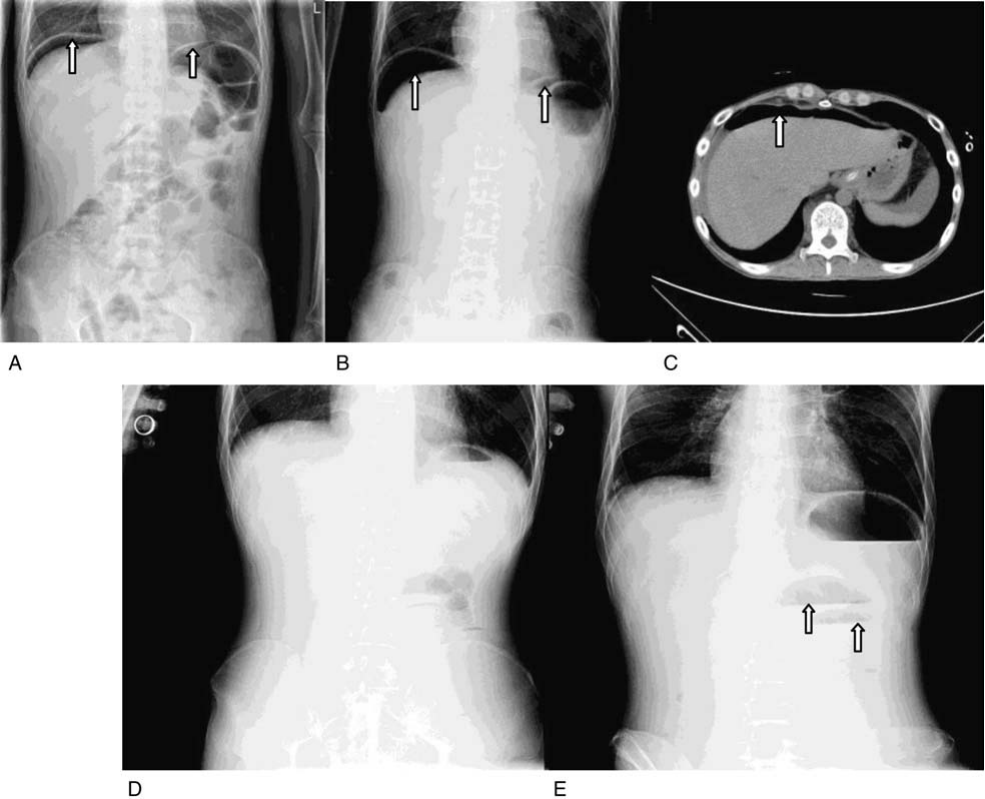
(A) An upright abdominal x-ray in a tertiary level hospital revealed bilateral moderate intraperitoneal free air. (B) An upright abdominal x-ray revealed bilateral massive intraperitoneal free air. (C) The image of abdominal CT showed free gas with a low diaphragm. (D) An upright abdominal x-ray did not show any free gas or liquid gas levels. (E) An upright abdominal x-ray showed visibly dilated intestines and gas-fluid levels.
